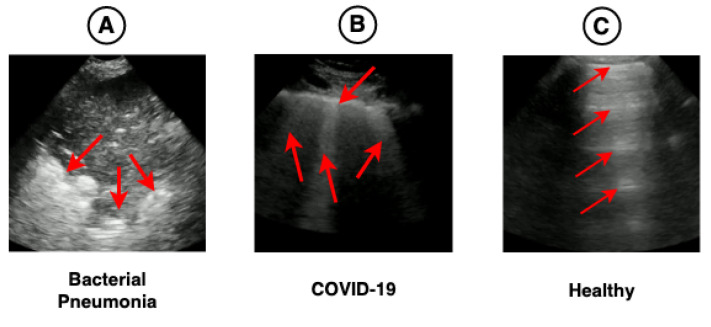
Image (A) shows a large consolidation area related to the diagnoses of bacterial pneumonia. Image (B) shows B-lines (vertical lines) and an irregular pleura line, both related to lung ultrasound (LUS) diagnosis of COVID-19. Image (C) is a lung with a healthy diagnosis, where there are A-lines (horizontal lines) and a regular pleural line. Red arrows were manually added to show the location of pathologies in the images.
